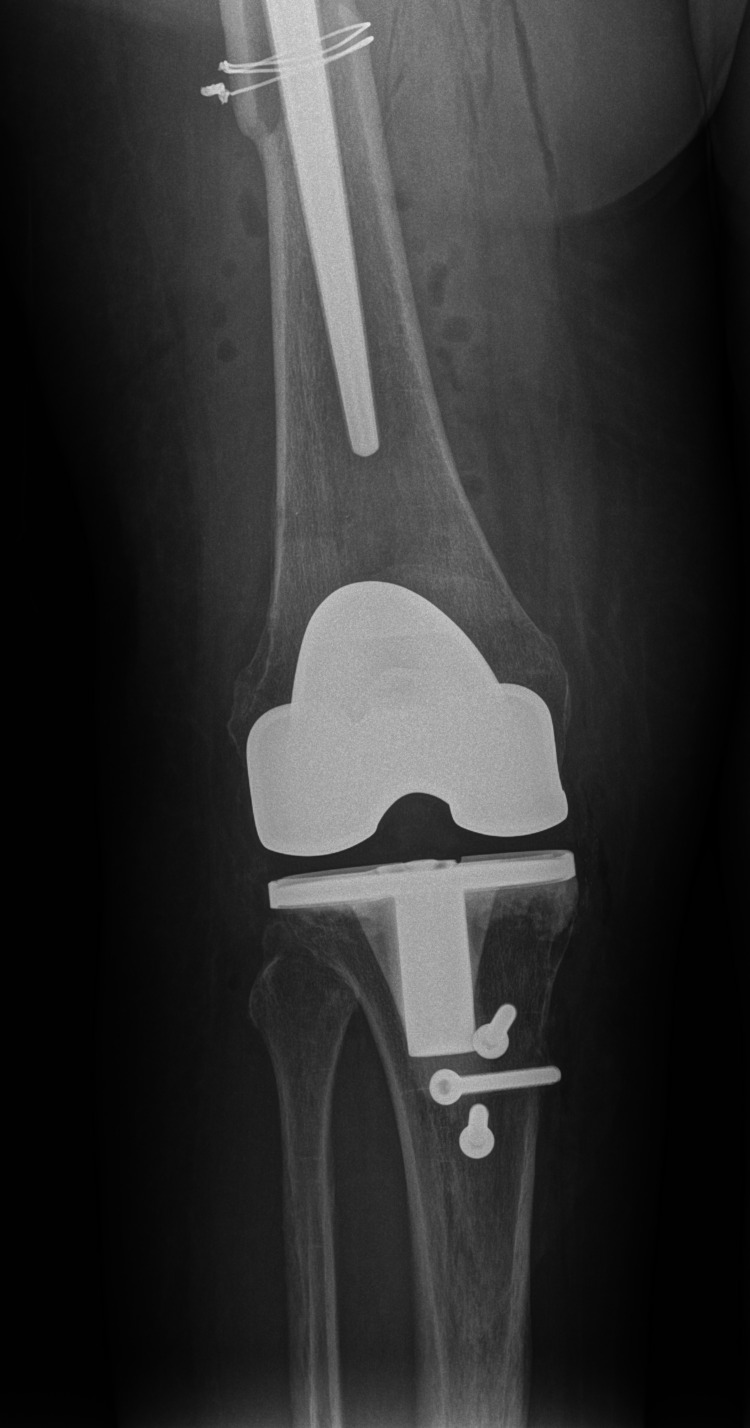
Anteroposterior X-ray of TKA and tibial tuberosity fixation TKA: total knee arthroplasty
